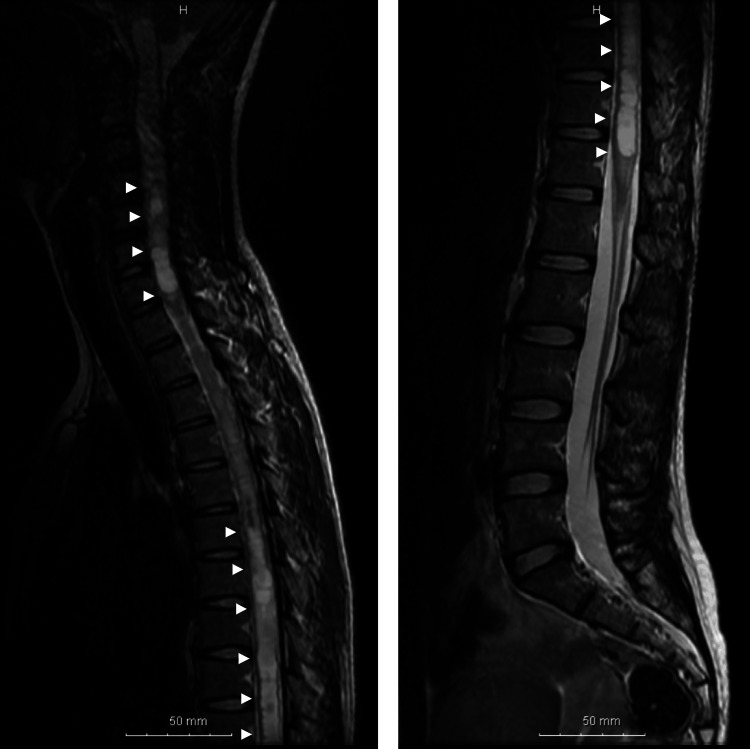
Magnetic resonance imaging of the spinal cord performed six months before pregnancy A T2-weighted image shows syringomyelia extending from the upper cervical region to T12.
